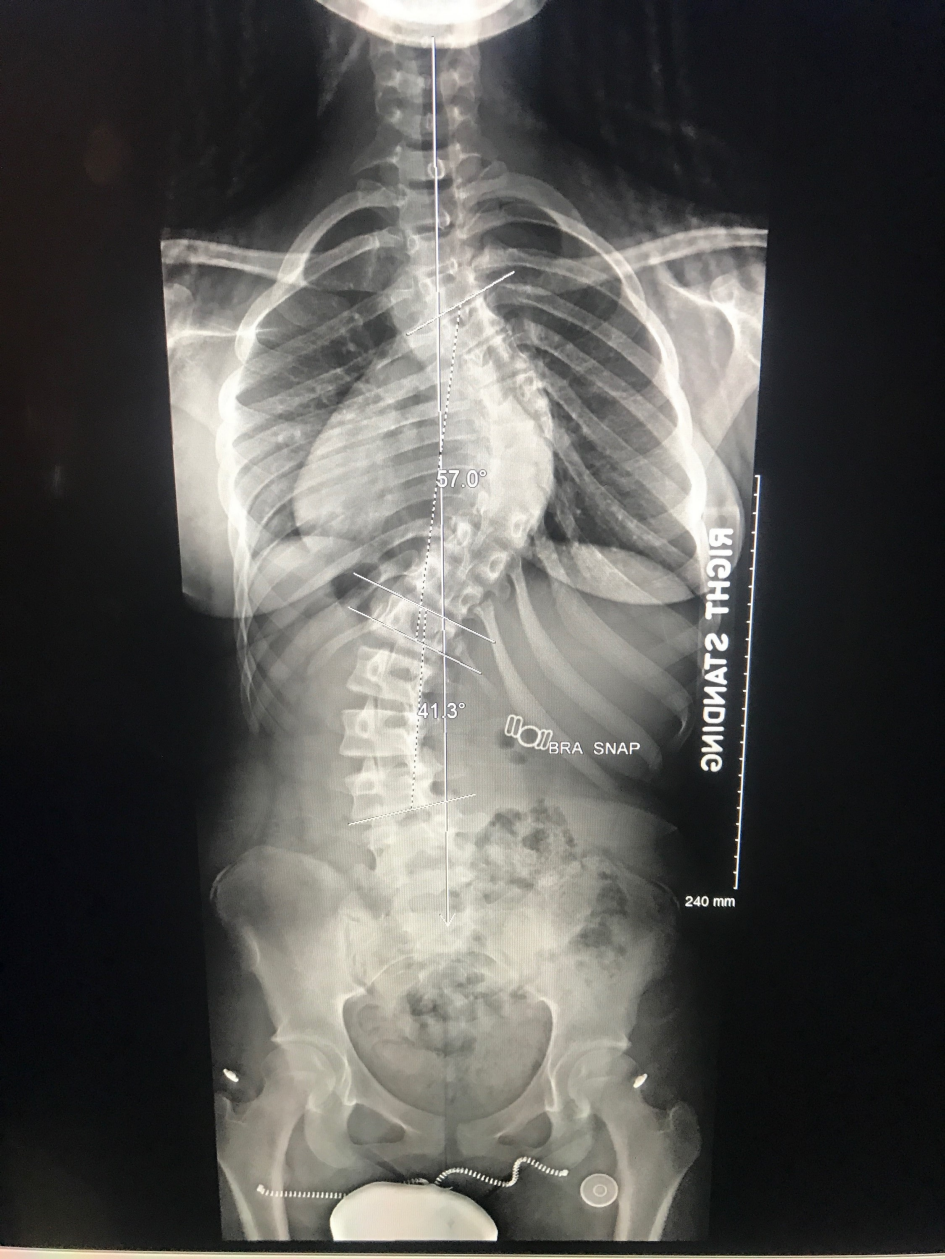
Preoperative posteroanterior (PA) 36-inch standing scoliosis radiograph.
